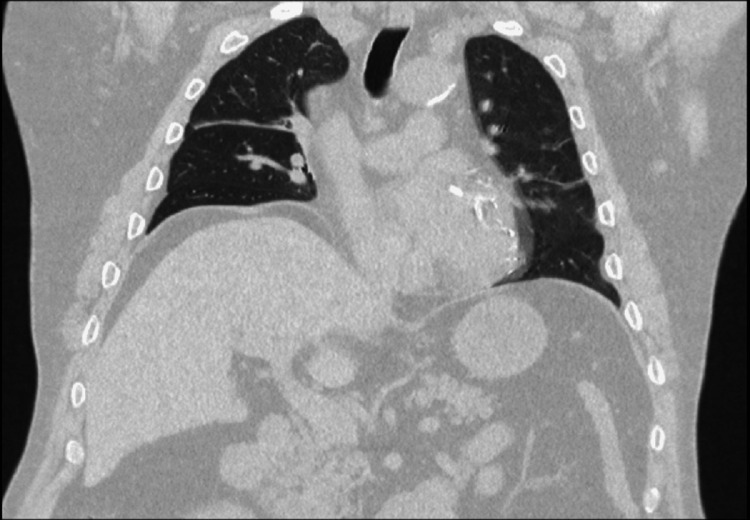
Coronal sections of chest CT scan. There are no structural lesions of the lung and liver parenchyma.
